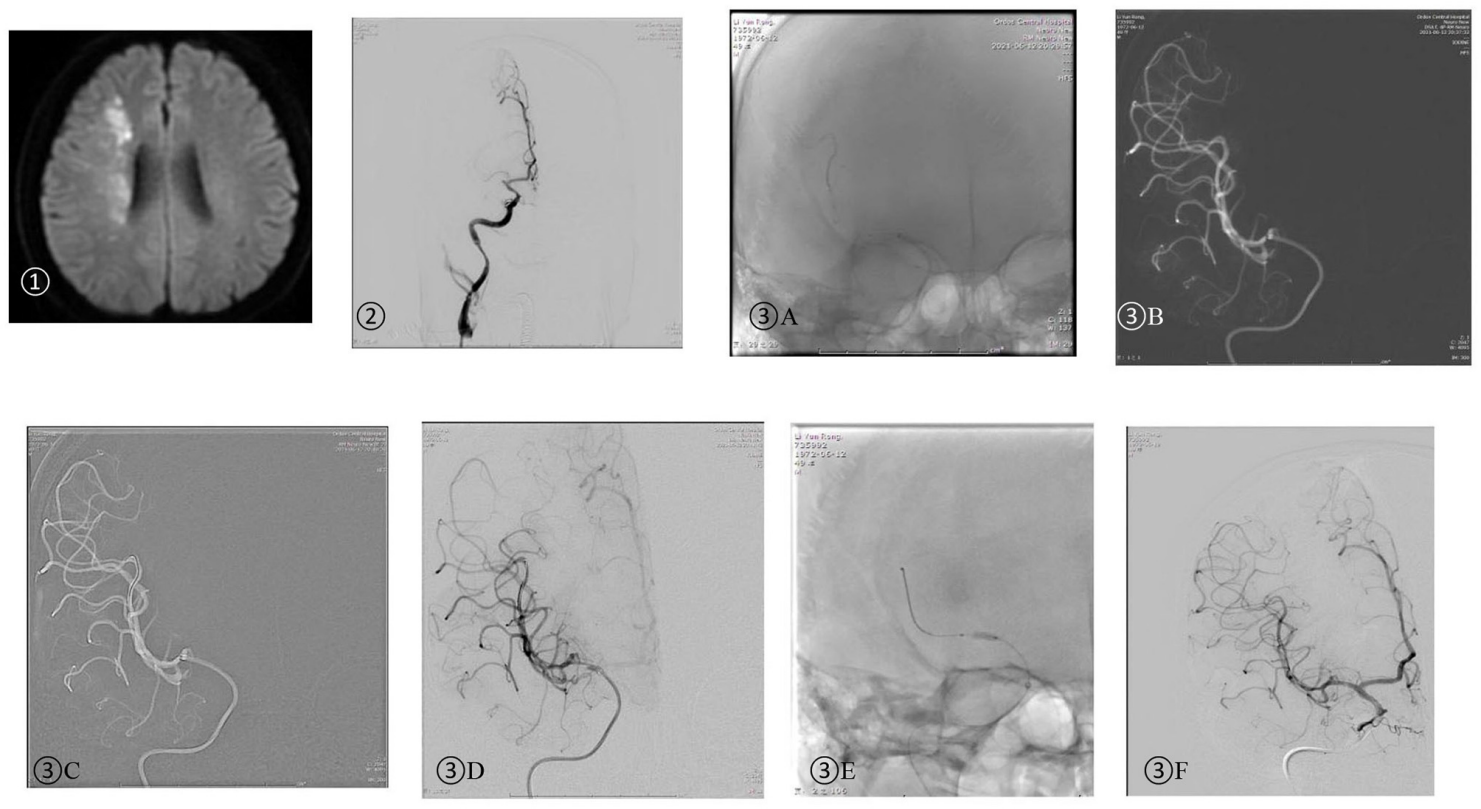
A 49-year-old man was admitted due to left limb numbness 12 h ago and left limb paralysis 6 h ago. NIHSS: 10.➀ Head DWI: watershed infarction of the right hemisphere; ➁ DSA: right MCA M1 initial part occlusion; ➂ SPACEMAN technique: (A) The aspiration catheter was placed near the occlusion segment and the stent retriever was released after verification of true vascular cavity by the microwire. (B) The aspiration catheter was placed through the thrombus over the stent retriever forming manual negative pressure. Once the aspiration catheter reached the end of the stent retriever (Figure 2➁), the stent retriever was then withdrawn under continuous negative pressure. (C) A 300 cm microwire was placed at MCA M2 distal segment under the guidance of the aspiration catheter. (D) Angiography was performed after the aspiration catheter was withdrawn proximal to the occlusion and showed severe M1 stenosis. (E) The balloon-expandable stent was delivered to the stenotic segment under the guidance of the aspiration catheter. (F) DSA performed after angioplasty showed complete recanalization.
